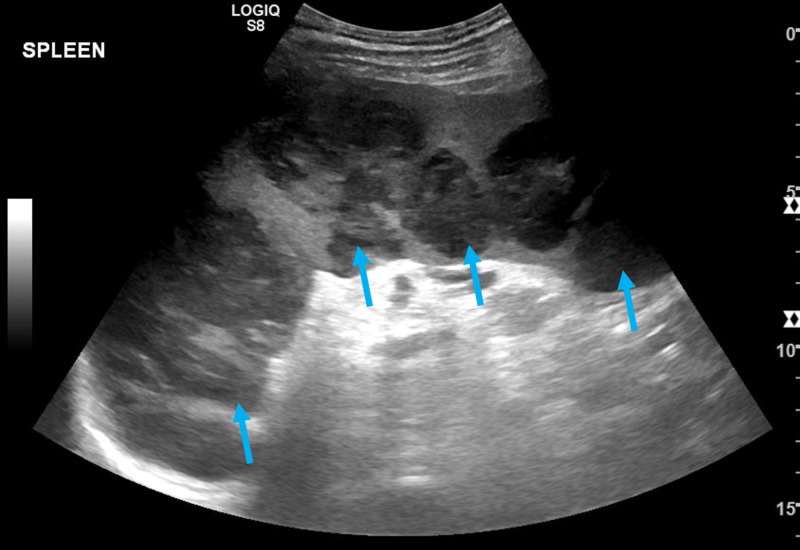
Abdominal ultrasound read as: “Splenic enlargement up to 18.5 cm in craniocaudal dimension. Multiple heterogeneous hypoechoic masses are noted throughout the spleen. The largest measures 9.6 x 7.8 x 8.9 cm. The masses demonstrate internal color Doppler flow.” Hypoechoic masses highlighted with arrows.
